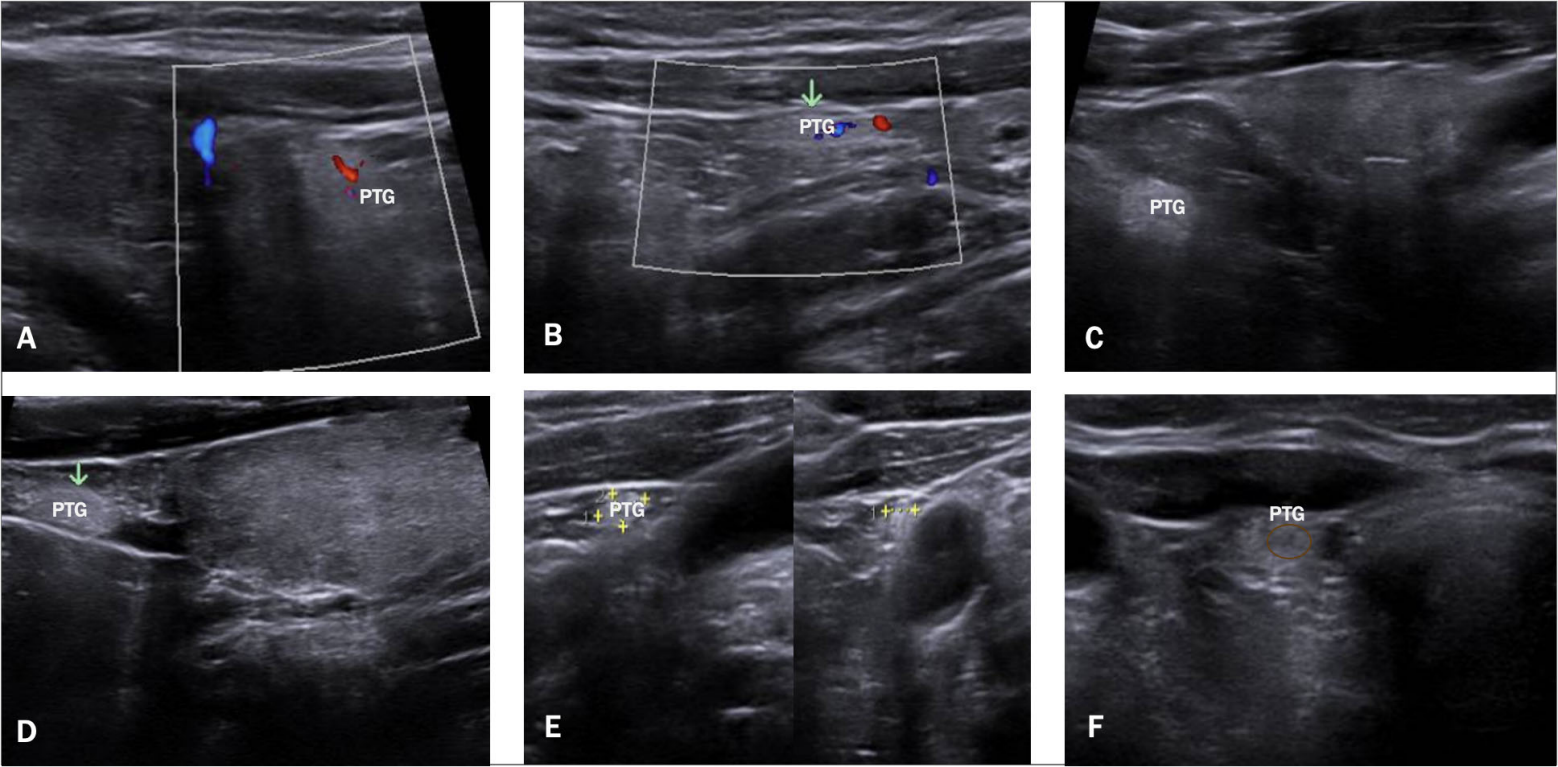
Ultrasound Images of PTGs. This figure displays a series of US images. (A, B) show a visible vascular hilum using color Doppler, (C, D) show normal PTGs with mild hyperechogenicity, (E) highlights the ectopic PTGs adjacent to the carotid artery, and (F) shows a normal PTGs with its visible hypoechoic hilum.
